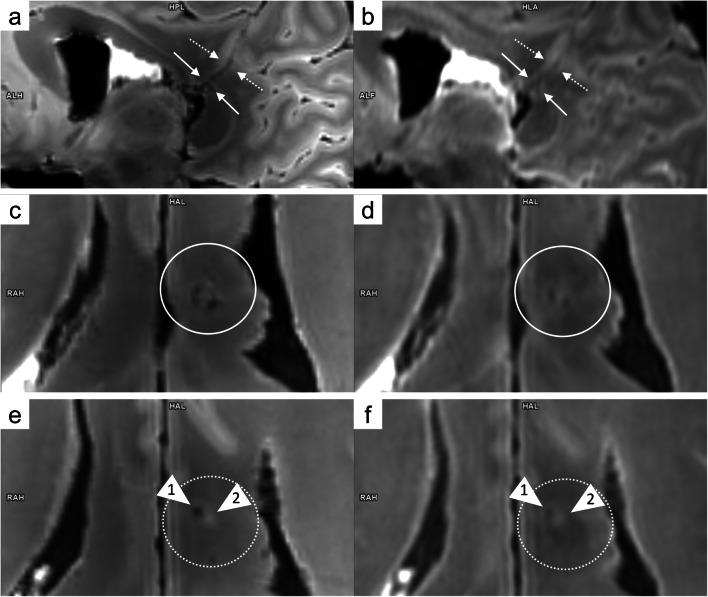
Comparison of 7 T T_2_-weighted images (a: sagittal view; c and e: transversal view) and 3 T T_2_-weighted images (b: sagittal view; d and f: transversal view) in case 3. The wound tract is identifiable on 7 T MRI (a: arrows) but difficult to delineate on 3 T MRI (b: arrows). In a cross-section of the bullet path (c and d: aligned according to the white arrows in a and b; e and f: aligned according to the white dashed arrow in a and b), 7 T allowed visualization of tiny injuries along the bullet path (c: white circle, hyper intense/dark structures) that were barely identifiable on 3 T (d: white circle). Furthermore, the tiny wound tract through the white matter was visible only on 7 T MRI (e: arrowhead 1). A small bright/hyper intense region was identified directly adjacent to the wound tract (arrowhead 2)
